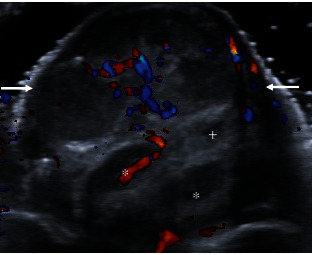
Grey scale ultrasound with Doppler demonstrating Doppler flow to the median raphe cyst (arrows). The corpora cavernosa (∗) and corpus spongiosum (+) are seen distinct from the cyst.
